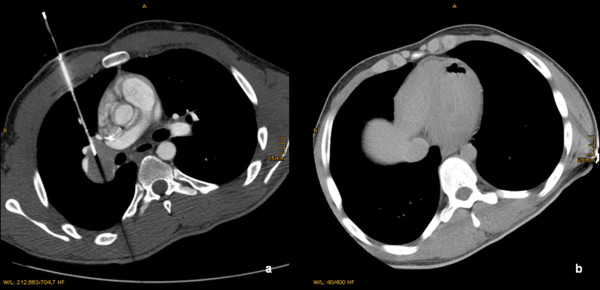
a and b: Transversal CT-slice of a 29 y/o patient during the biopsy of a tumor located in the right hilum of the lung (a). The CT-Scan after biopsy showed a SAE in the apex of the left ventricle (b), which was treated immediately. There were no clinical sequelae.
